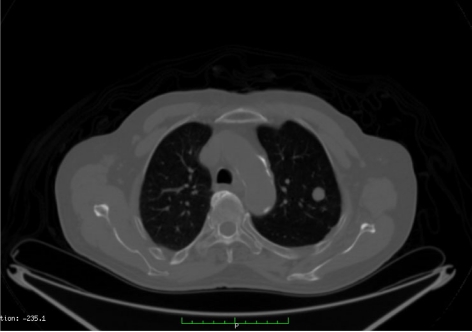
CT image of single lung metastasis pre-RFA.
